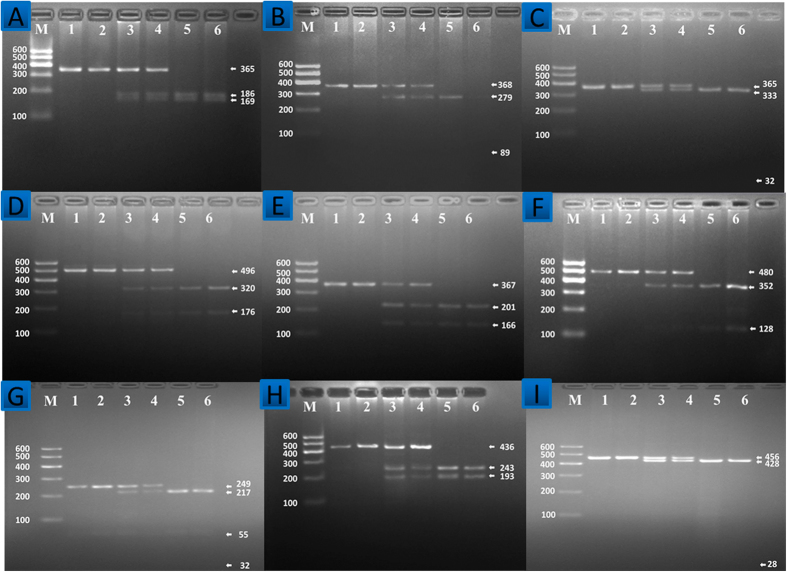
Agarose gel electrophoresis (2%) of genotyping of the *DOCK7*, *PCSK9* and *GALNT2* SNPs. Lane M: DNA ladder100bp. The genotypes of 9 SNPs were as follow: (A) *DOCK7* rs1168013: CC (Lanes 1 and 2, 365-bp); CG (lanes 3 and 4, 365-, 186- and 169-bp); and GG genotype (lanes 5 and 6, 186- and 169-bp). (B) *DOCK7* rs10889332: TT (lanes 1 and 2, 368-bp); CT (lanes 3 and 4, 368-, 279- and 89-bp); and CC genotype (lanes 5 and 6, 279- and 89-bp). (C) *PCSK9* rs615563: AA (lanes 1 and 2, 365-bp); AG (lanes 3 and 4, 365-, 333-, 32-bp); and GG genotype (lanes 5 and 6, 333- and 32-bp). (D) *PCSK9* rs7552841: TT (lanes 1 and 2, 496-bp); CT (lanes 3 and 4, 496-, 320- and 176-bp); and CC genotype (lanes 5 and 6, 320- and 176-bp). (E) *PCSK9* rs11206517: TT (lanes 1 and 2, 367-bp); GT (lanes 3 and 4, 367-, 201- and 166-bp); and GG (lanes 5 and 6, 201- and 166-bp). (F) *GALNT2* rs1997947: AA (lanes 1 and 2, 480-bp); AG (lanes 3 and 4, 480-, 352- and 128-bp); and GG genotype (lanes 5 and 6, 352- and 128-bp). (G) *GALNT2* rs2760537: TT (lanes 1 and 2, 249- and 55-bp); CT (lanes 3 and 4, 249-, 217-, 55- and 32-bp); and CC genotype (lanes 5 and 6, 217-, 55- and 32-bp). (H) *GALNT2* rs4846913: AA (lanes 1 and 2, 436-bp); AC (lanes 3 and 4, 436-, 243-and 193-bp); and CC genotype (lanes 5 and 6, 243- and 193-bp). (I) *GALNT2* rs11122316: AA (lanes 1 and 2, 456-bp); AG (lanes 3 and 4, 456-, 428- and 28-bp); and GG genotype (lanes 5 and 6, 428- and 28-bp). The less than 90-bp fragment was invisible in the gel owing to its fast migration speed.
